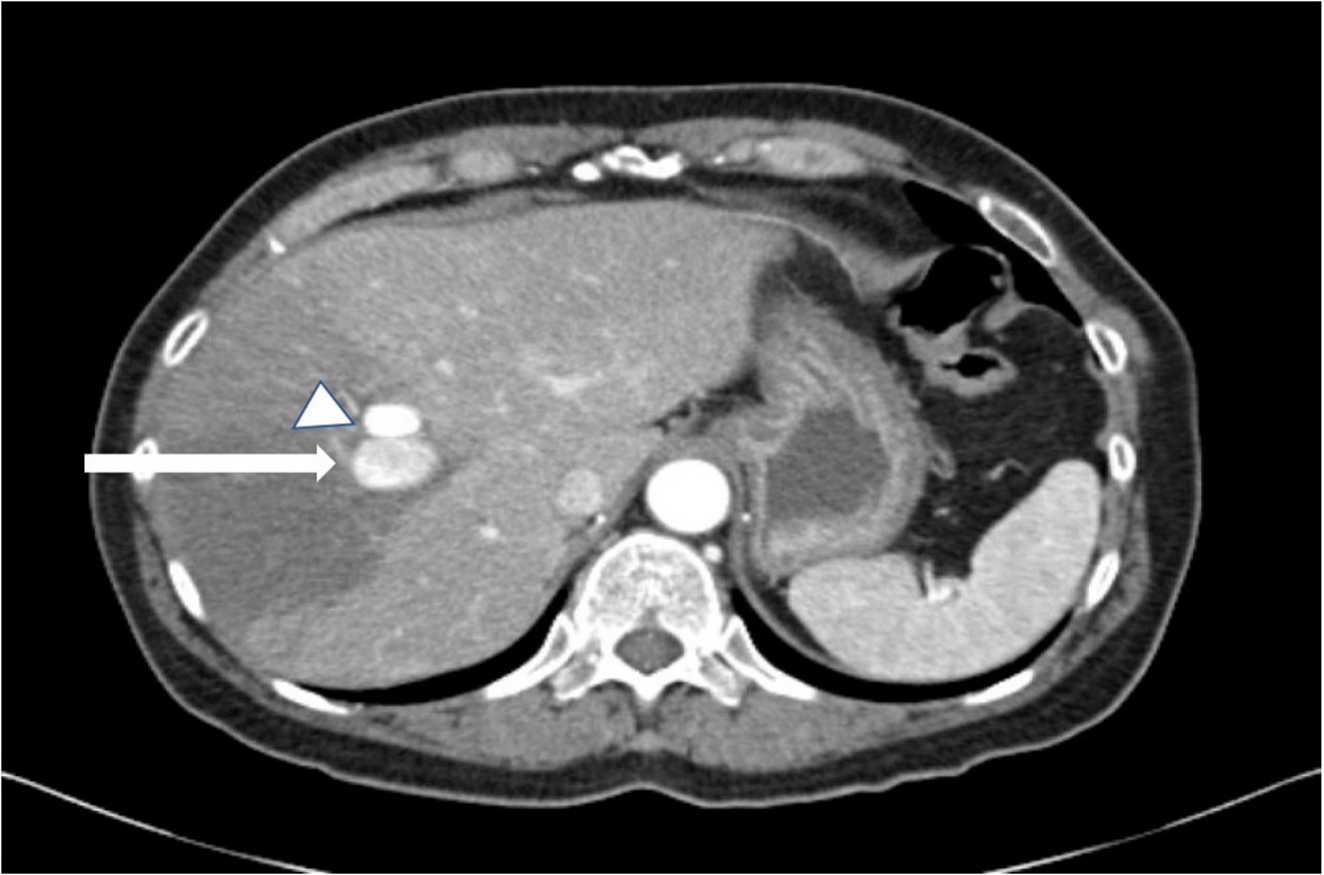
Axial contrast‐ enhanced computed tomography angiography showing pseudoaneurysms in right hepatic artery (arrow and arrow head).
